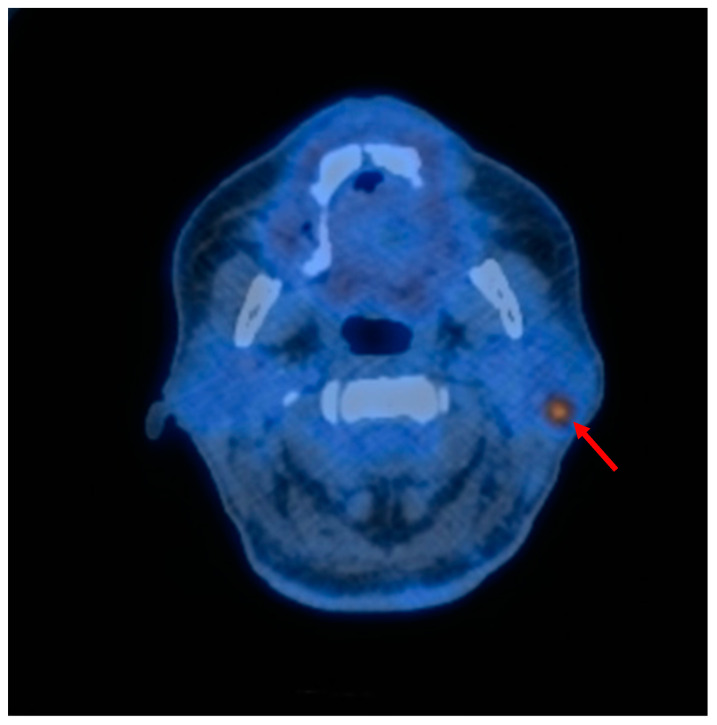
PET/CT scan: horizontal view with WT in the tail of the left parotid gland in a patient following resection of squamous cell carcinoma of the floor of the mouth. Abnormal, high FDG uptake on PET/CT in WT may be a misleading indication of lymph node metastasis (red arrow).
